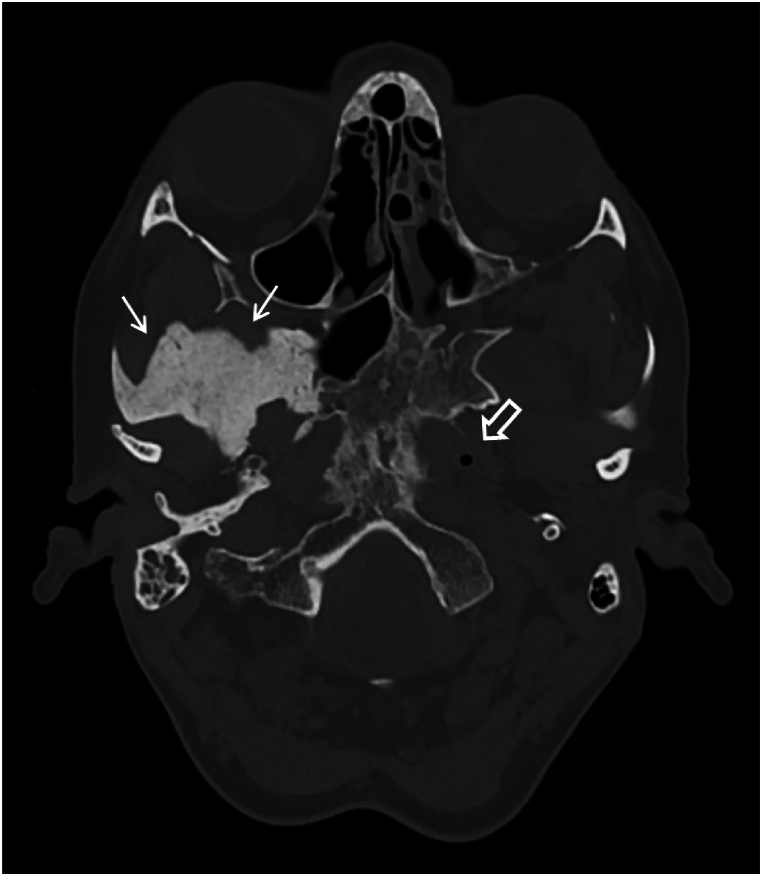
Axial CT image showing evident right temporal bone hyperostosis as part of the intra-osseous meningioma (arrows). Air in the open Eustachian tube on the left (open arrow) and no air in the closed one on the right.
